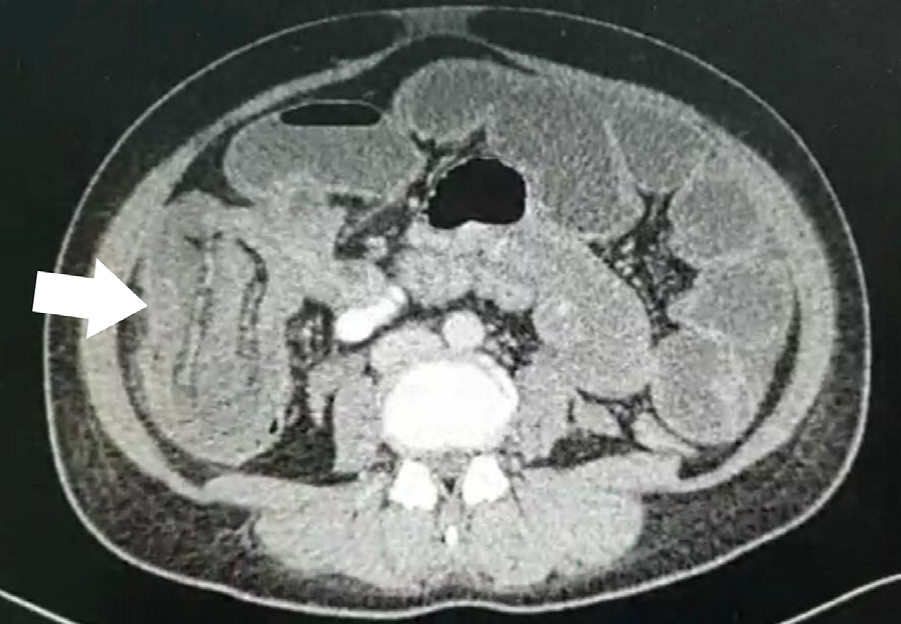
CT scan showing classical feature of elongated, sausage-shaped mass (arrow) in longitudinal view which represents an intussusception.
